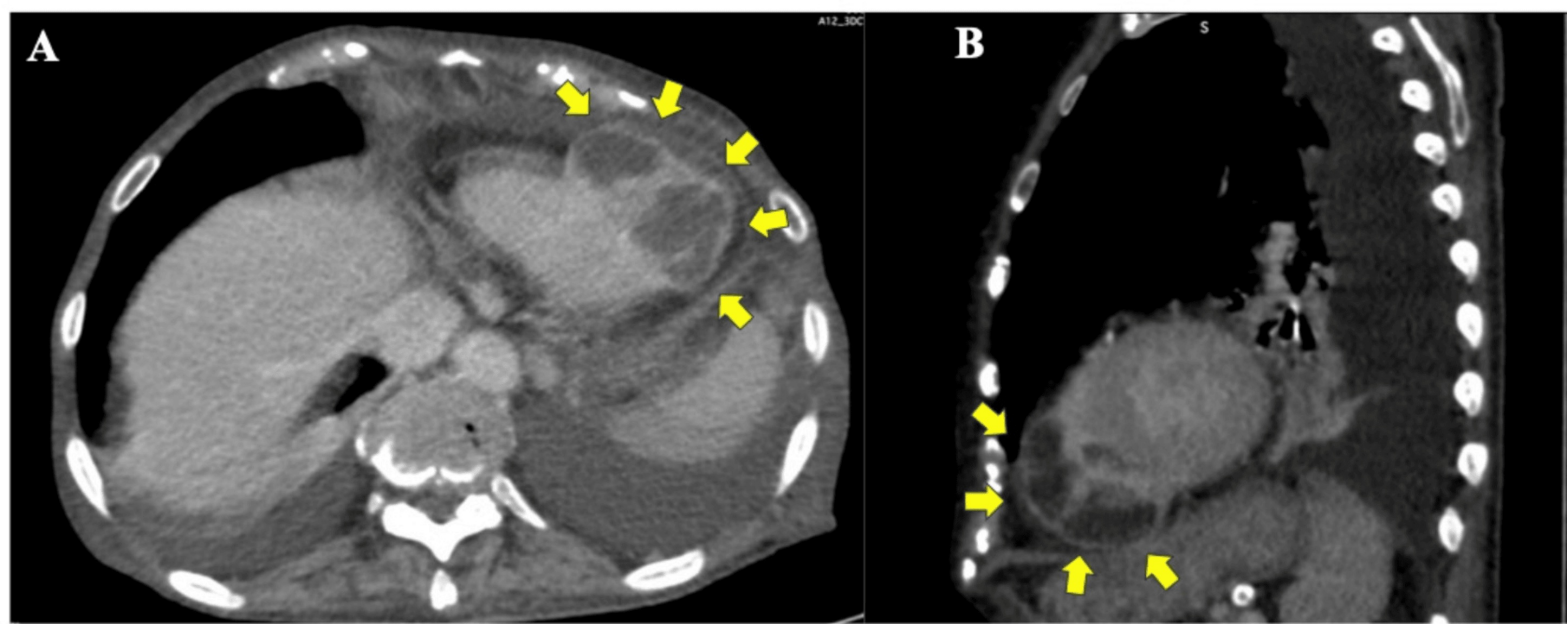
Enhanced computed tomography images (A: horizontal section; B: sagittal section). A 53-mm × 40-mm tumor with contrast enhancement around its periphery is connected to the right ventricle (arrow).
